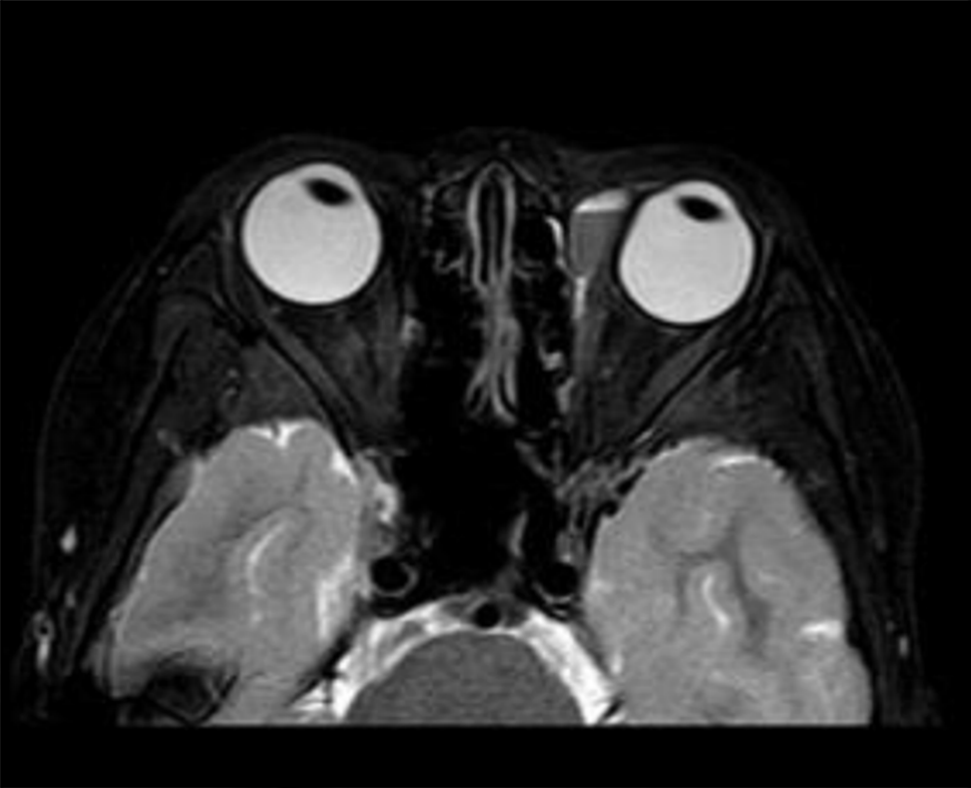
A 10-year-old girl presented with a left periorbital LMs with intracystic hemorrhage on MRI, accompanied by mild compression of the globe
